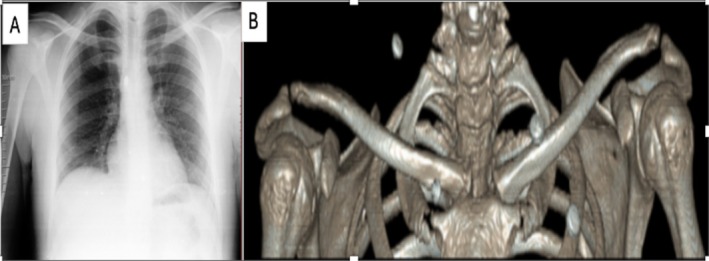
Initial imaging demonstrating posterior sternoclavicular joint dislocation. (A) Anteroposterior chest radiograph showing asymmetry of the medial clavicles with depression of the right sternoclavicular joint, raising suspicion for posterior displacement. (B) Three‐dimensional computed tomography (3D‐CT) confirming posterior sternoclavicular joint dislocation of the right medial clavicle without associated fracture.
